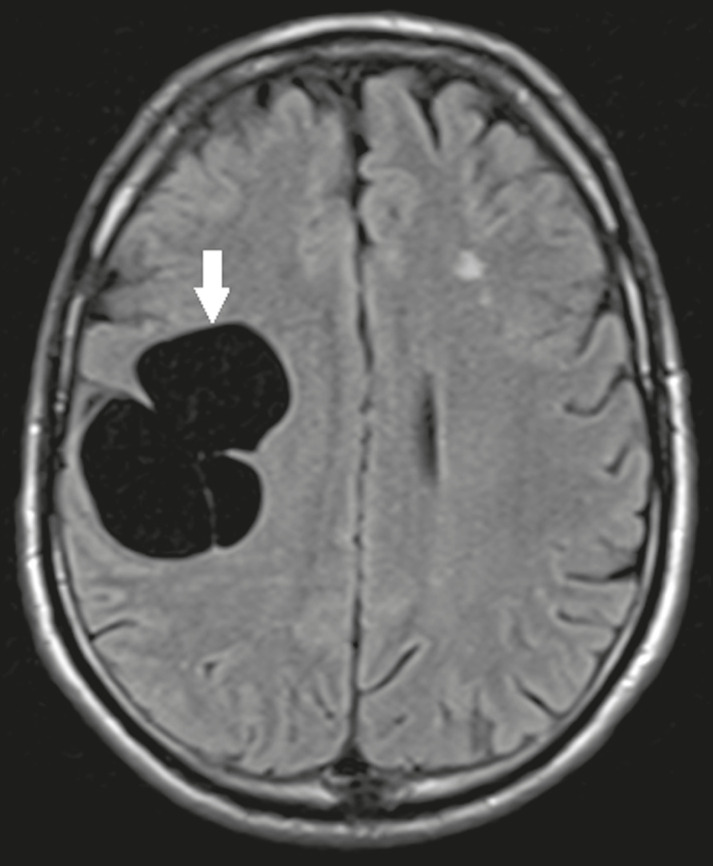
Neuroglial cyst. Axial FLAIR MRI sequence showing a cyst-like formation in the white matter of the right frontal lobe with signal intensity similar to that of the CSF and without surrounding edema (arrow).
